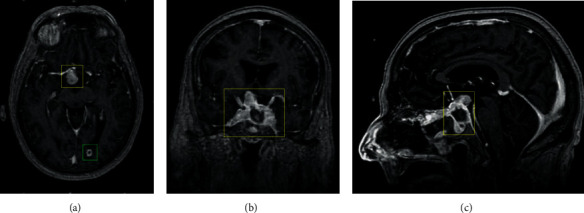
Radiographic imaging of tumor progression postoperative. T1-weighted sequence magnetic resonance imaging with double contrast presented in axial, coronal, and sagittal planes, respectively. The mass has undergone subtotal resection (a–c). The left occipital lesion is stable (a). The suprasellar lesion measures 2.7 x 1.4 x 2.0 cm with total volume calculated at 4.6 mL. Yellow boxes highlight the tumor of interest. Green box highlights occipital lesion.
